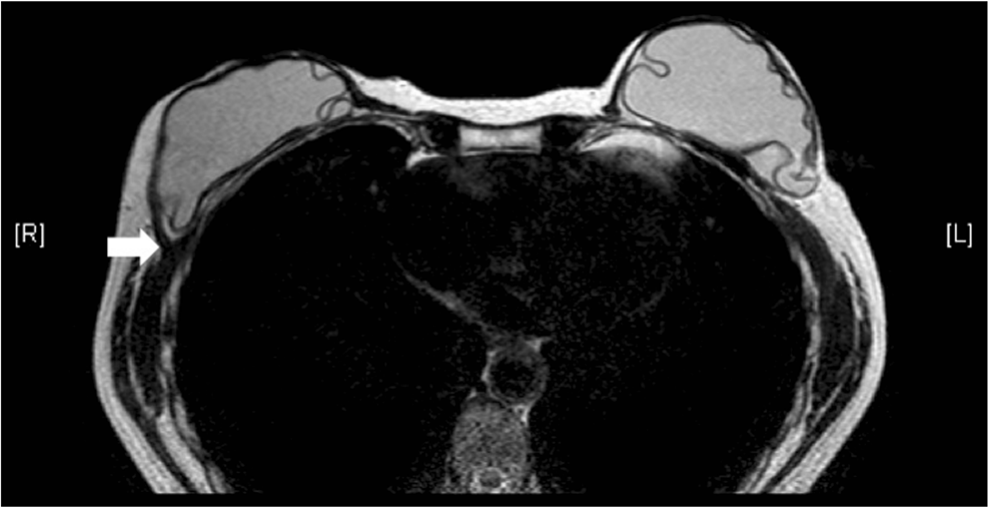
Retropectoral position of implants. T2-weighted MR image on axial plane demonstrates the retropectoral position of the right silicone gel-filled implant. Note the right pectoral muscle (arrow) is split by the implant
